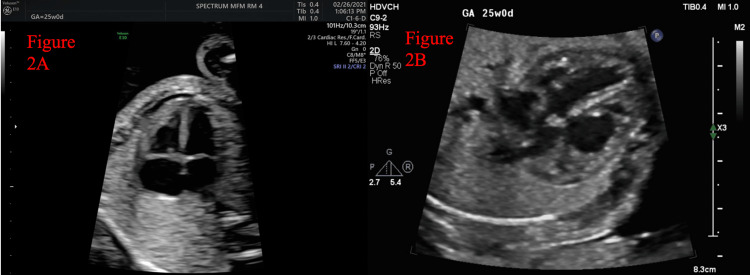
Apical four-chamber and transverse view at 25 weeks of gestational age Apical four-chamber view (A) and transverse view (B) demonstrating an absence of ventricular hypertrophy, endocardial fibroelastosis (EFE), or pericardial effusion at the initial echocardiogram at 25 weeks of gestation
